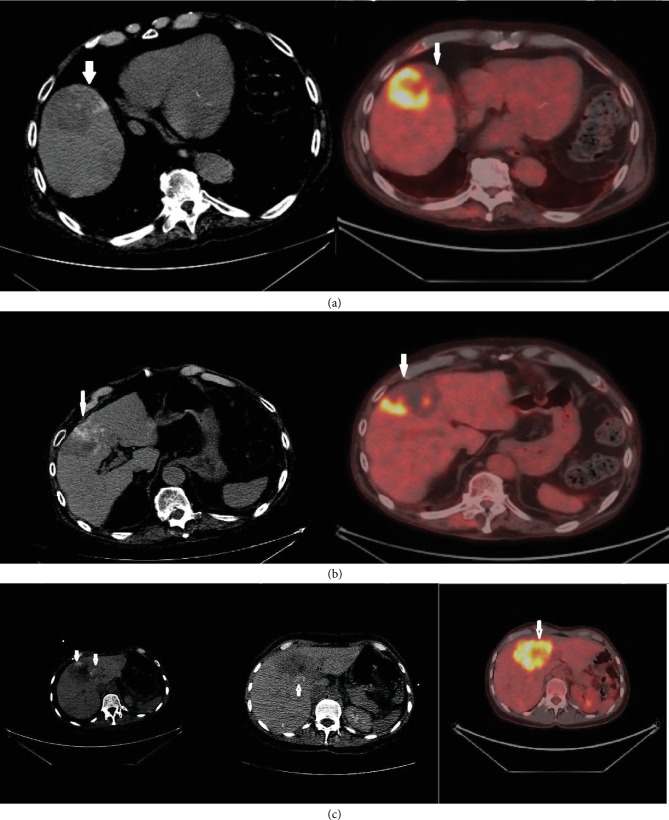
(a, b) The column on the left demonstrates post embolization contrast retention on CT (arrows). The PET-CT images on the right demonstrates corresponding hypometabolic areas. Findings are in keeping with significant overlap. (c) The CT on the left was obtained after DEBIRI treatment to the left lobe of the liver. The middle CT demonstrates contrast retention after the DEBIRI treatment to the right lobe of the liver. There is no corresponding hypometabolic area on PET-CT. Findings would be in keeping with a “no match.”
